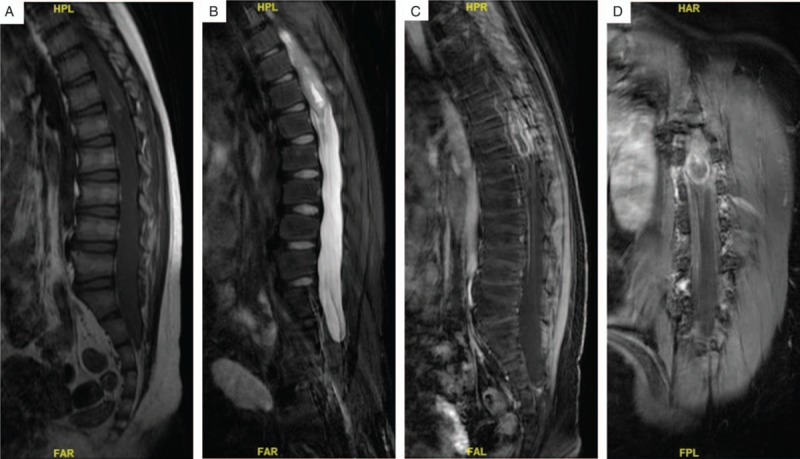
(A, B) T1-weighted and T2-weighted sagittal magnetic resonance imaging (MRI) without contrast shows an intramedullary lesion extended from T11 to L1. (C) T1-weighted sagittal MR image with contrast reveals heterogeneous enhancement of the conus lesion. (D) T1-weighted coronal MR image with contrast.
